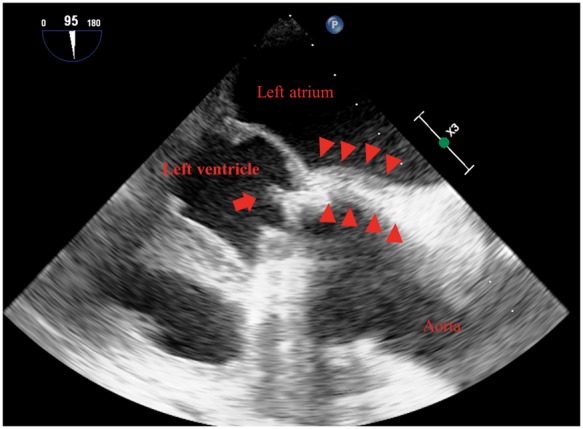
Transoesophageal echocardiography showing a thickened area along the aortic root behind the non-coronary sinus of Valsalva (arrowheads) and a mobile echogenic mass attached to the prosthetic valve leaflet (arrow).
